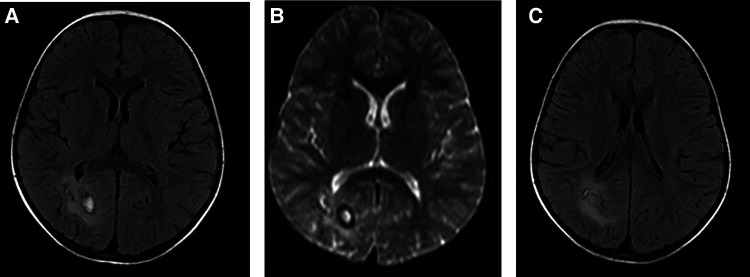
A 5-year-old girl visited our hospital due to limb movement disability. Brain MRI showed the lesion and its surrounding edema (A, B), and tunnel-like transitional signs (C).
